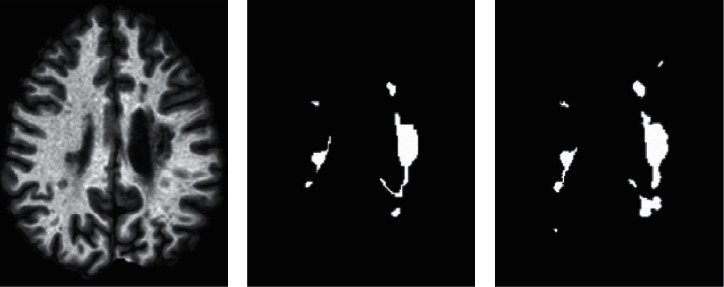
Manual segmentation of MS lesions: (a) T1w MRI, (b) manual segmentation by rater 1, and (c) manual segmentation by rater 2.
